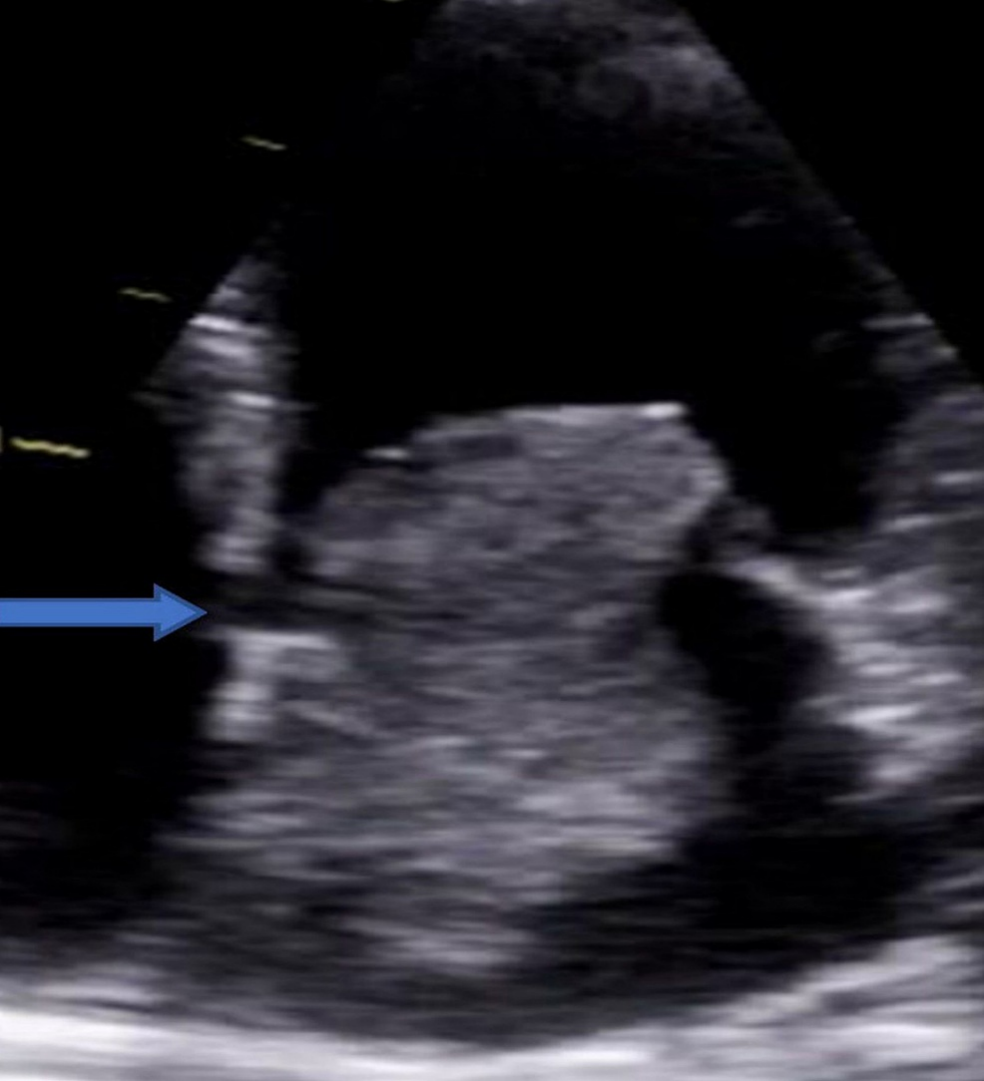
Echocardiography apical four-chamber view showing left atrial myxoma
